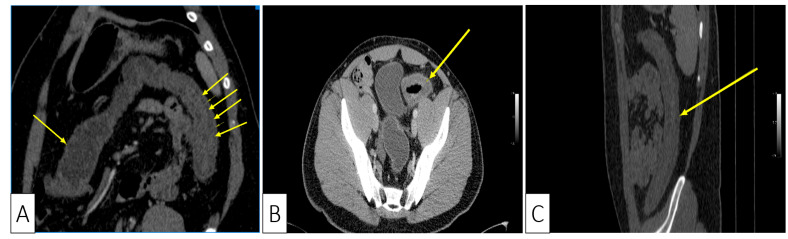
Abdominal CT showing: (A) Coronal plane, oblique reconstruction image showing the difference between the normal wall (single arrow at the transverse colon) and the thickened pathological wall of the left colon (multiple arrows); (B) Axial plane, venous phase, showing stratified wall thickening of the proximal sigmoid; (C) Sagittal plane, showing the left colonic involvement with symmetrical wall thickening.
